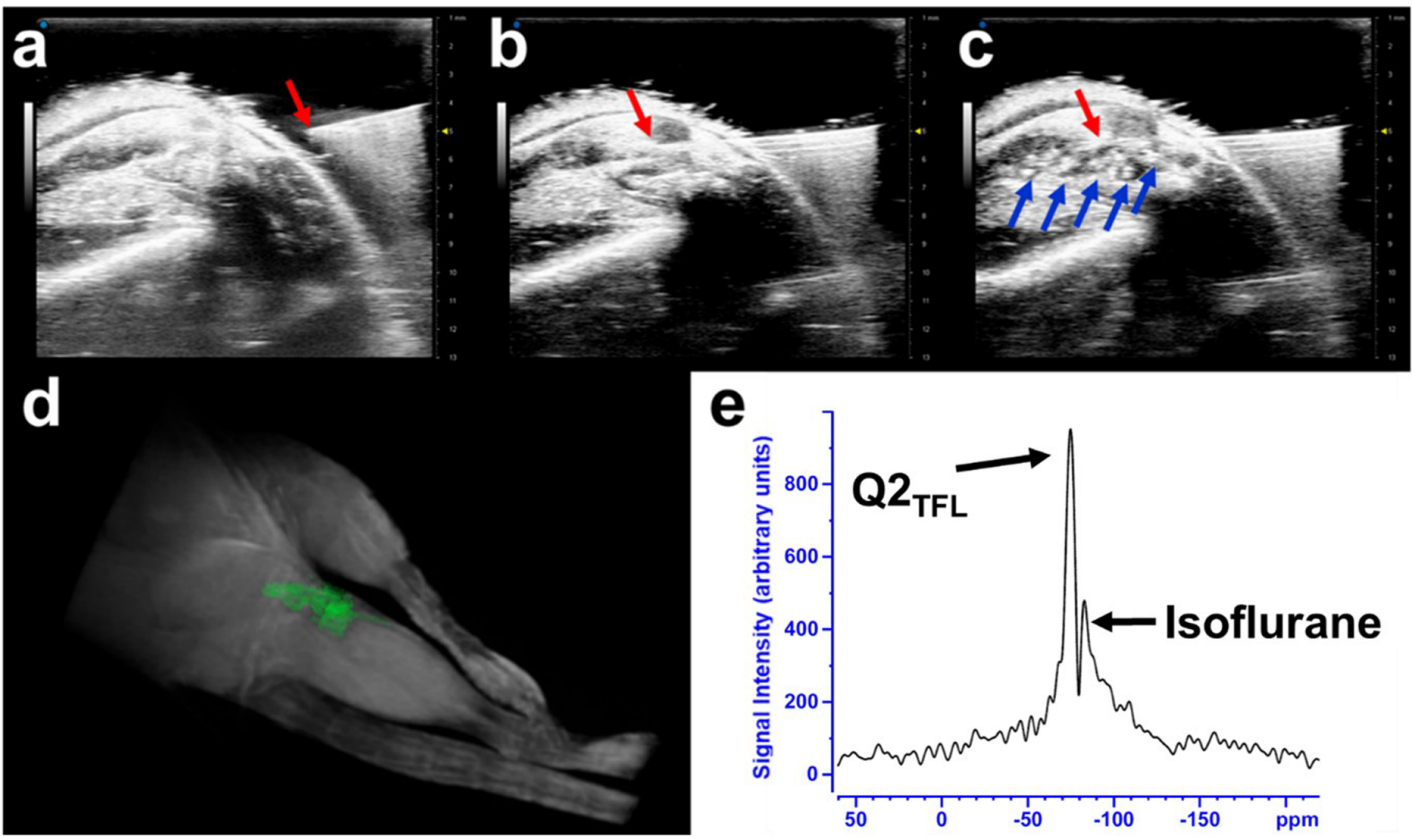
Ultrasound guided injection imaging: (a) Sagittal view
